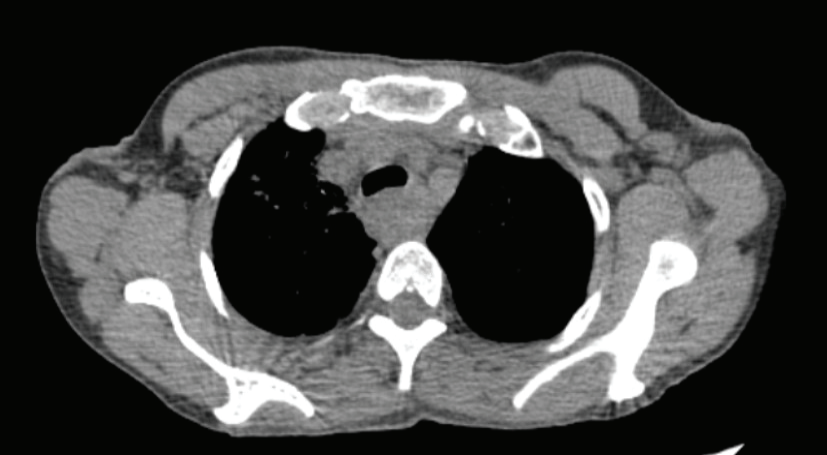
Axial CT scan images of the patient taken at the time of the baseline diagnostic scan.
